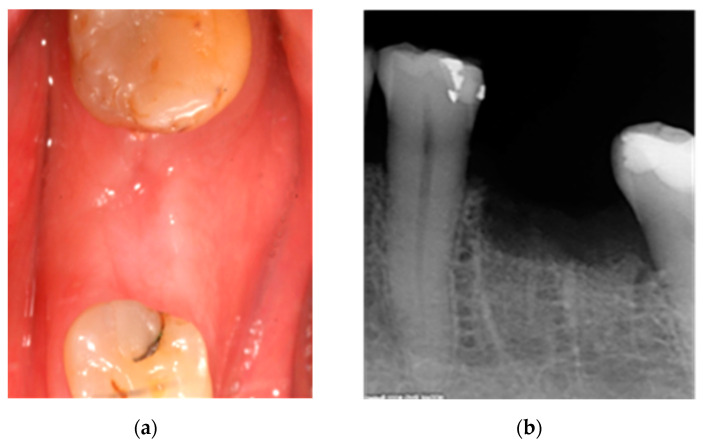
(a) Clinical aspect and (b) X-ray of the affected area 12 months after treatment.
